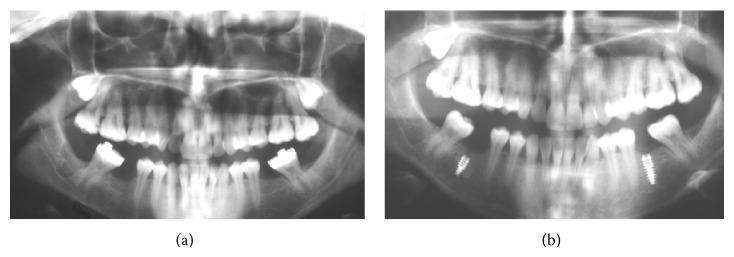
(a) Presurgical radiograph, (b) 6-month follow-up radiograph with (<8 mm) on the right and (8 mm) on the left side of the same patient.
